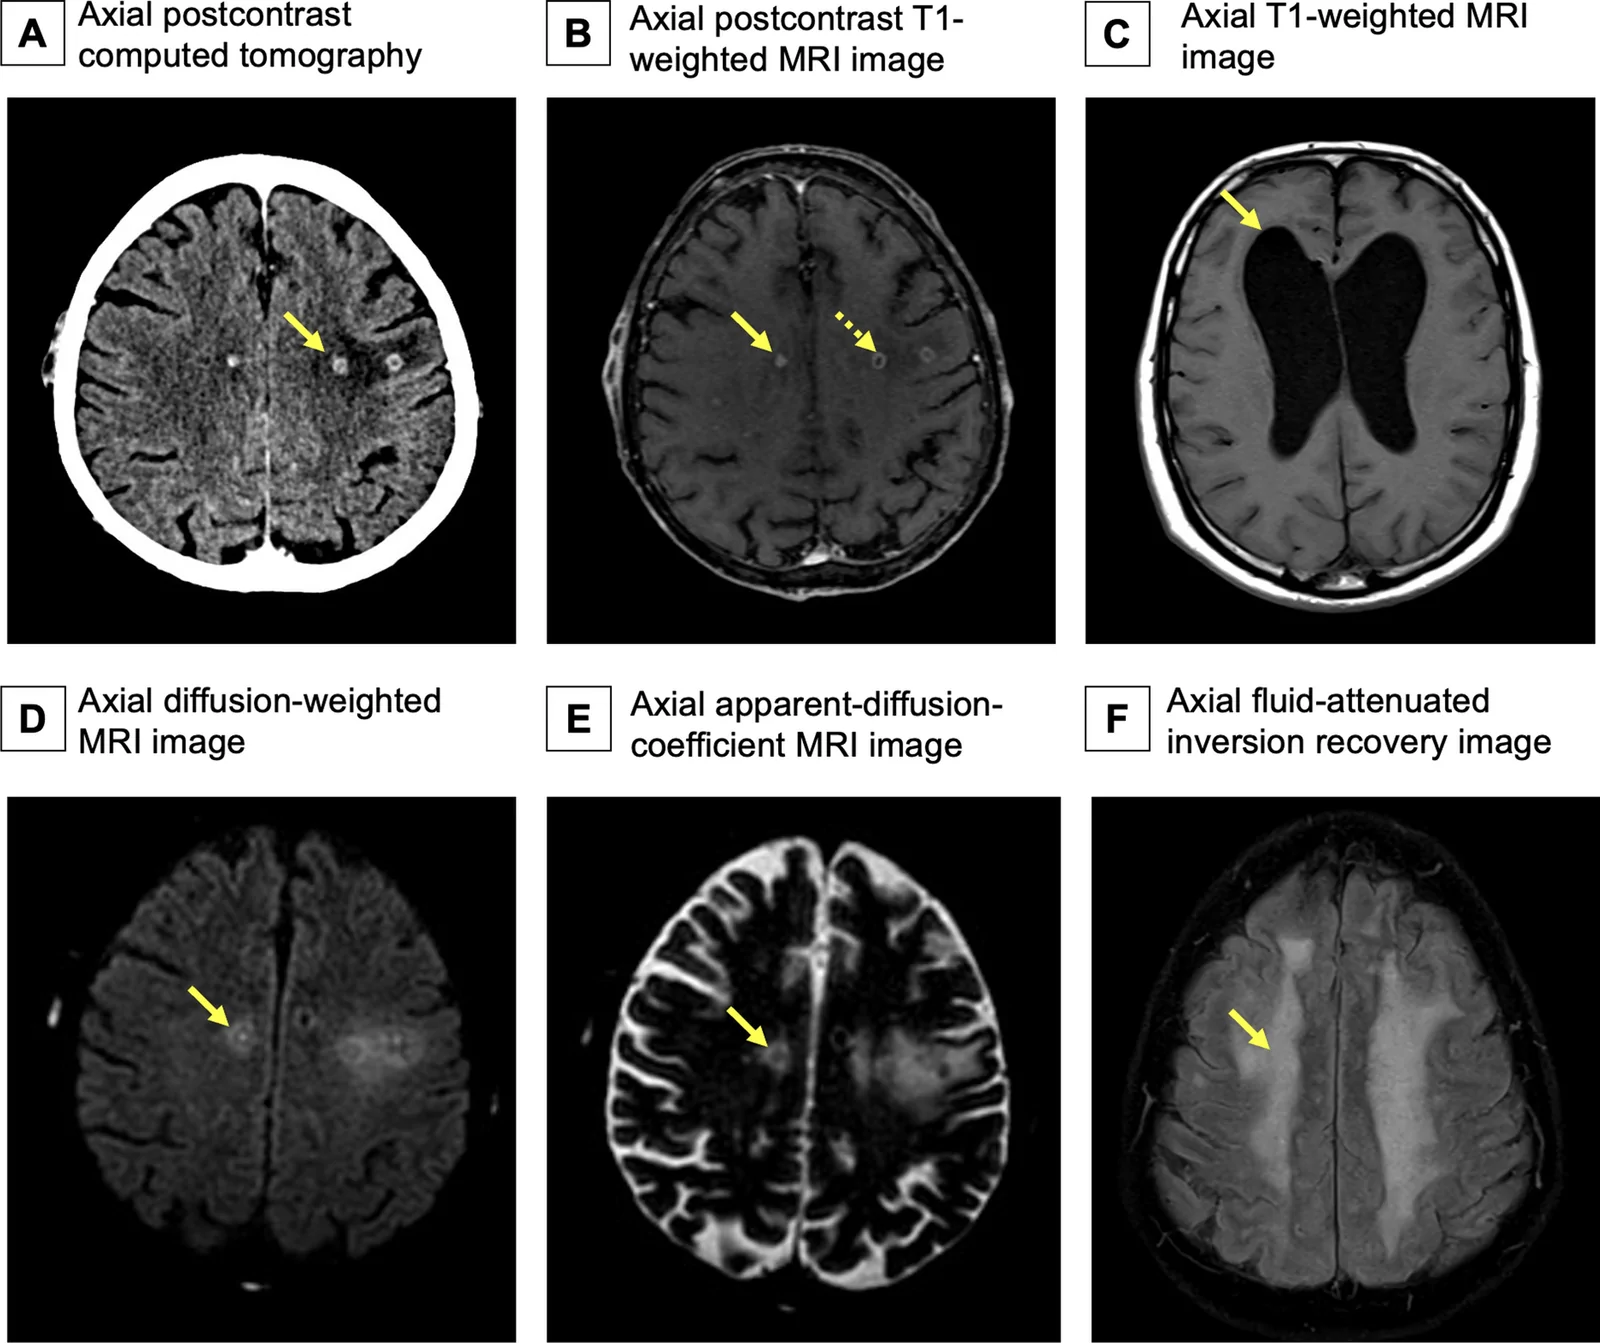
Neuroimaging findings in central nervous system candidiasis. Axial postcontrast computed tomography (A) and T1-weighted magnetic resonance (B) images show small nodular and ring-enhancing lesions in the frontal lobe. Axial diffusion-weighted imaging (D) and apparent-diffusion-coefficient (E) show restricted diffusion consistent with brain abscess. An axial T1-weighted magnetic resonance image shows hydrocephalus (C). An axial fluid-attenuated inversion recovery (FLAIR) image demonstrates bilateral white matter hyperintensities, consistent with leukoencephalopathy (F)
